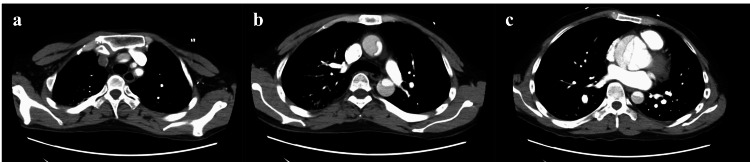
Contrast-enhanced computed tomography angiography of the chest (a) Dissection involving left common carotid artery, left vertebral artery, and brachiocephalic artery; (b) Dissection of ascending aorta and descending aorta with false cavity unstained; (c) Severely dilated Valsalva sinus with contrast entering a false cavity
